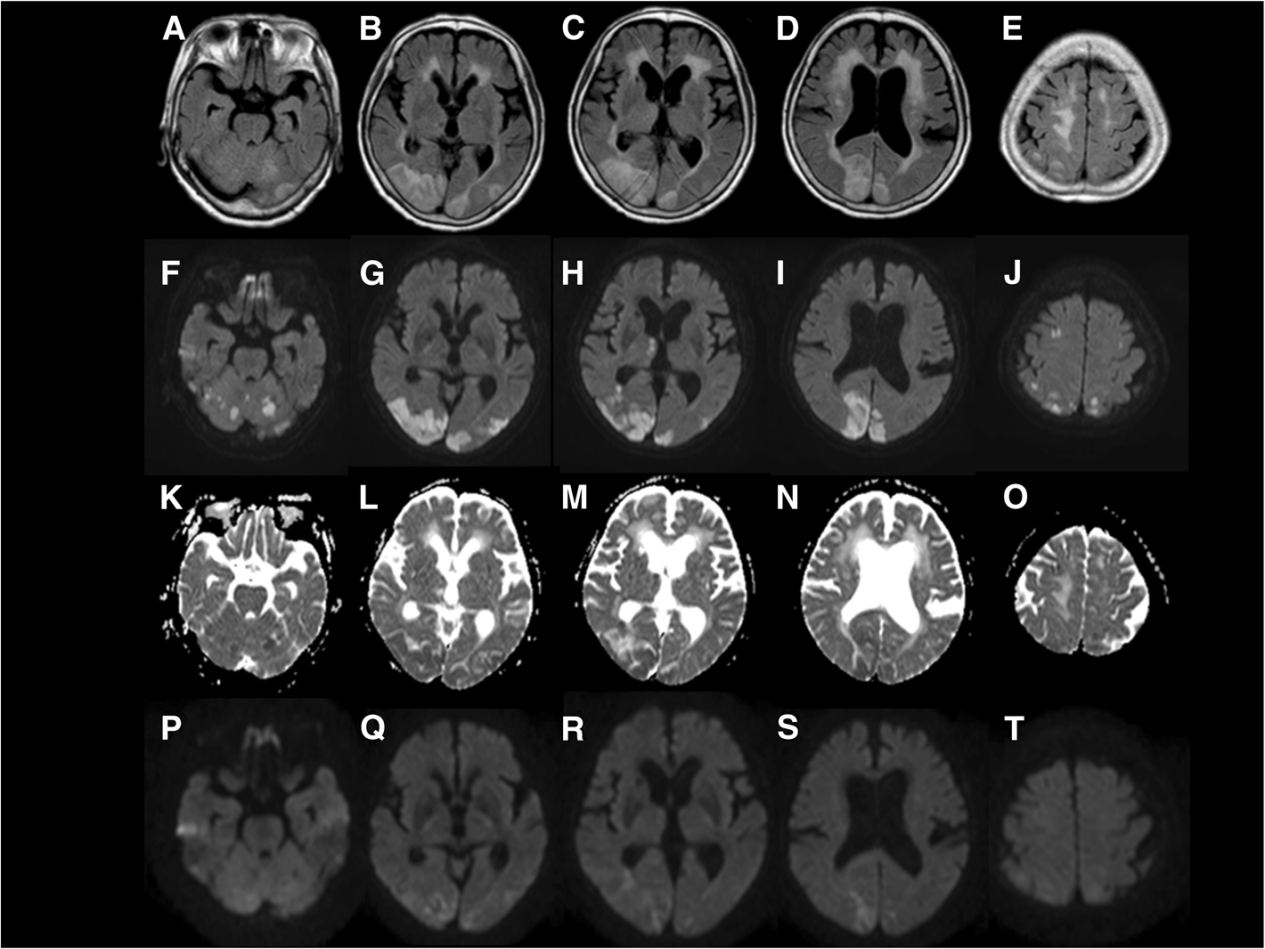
On admission day 2, axial fluid-attenuated inversion recovery (FLAIR) images show abnormal hyperintense areas bilaterally in the cerebellar hemispheres, watershed regions, and white and grey matter of the occipital and occipitoparietal lobes. Axial FLAIR images show hyperintense areas in white matter predominating in the periventricular region indicating leukoaraiosis (a, b, c, d, e). Axial diffusion weighted image (DWI) images show hyperintense areas in these lesions and the right thalamus (f, g, h, i, j). Apparent diffusion coefficient (ADC) map shows low signal intensities in the bilateral cerebellar hemispheres and watershed regions (k, o). ADC map shows low signal intensities in the cortical and subcortical regions with small areas of high signal intensity in the surrounding area (l, m, n). These image patterns indicate that the lesions were damaged by extensive cytotoxic edema with restricted vasogenic edema. Most hyperintense areas on DWI on 2 days after admission disappeared by 7 days after admission (p, q, r, s, t)
